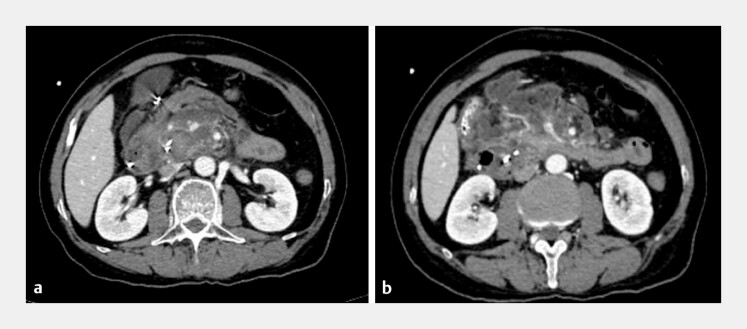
Enhanced computed tomography reveals multiple hypervascular spots in the pancreatic head and early visualization of the portal vein and superior mesenteric vein.
